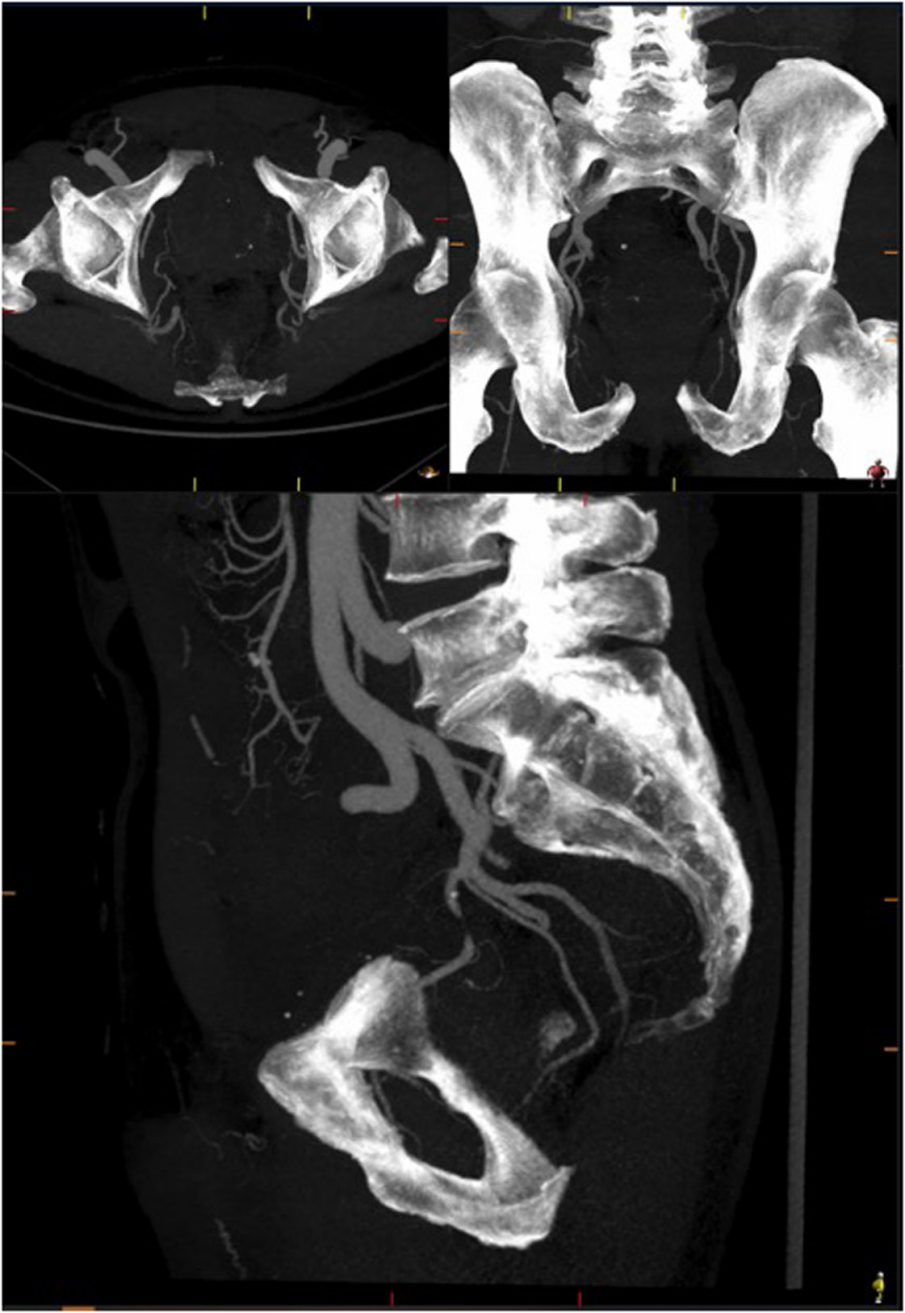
Planning computed tomography angiogram to identify the origin of the prostate arteries and facilitate procedural planning.
